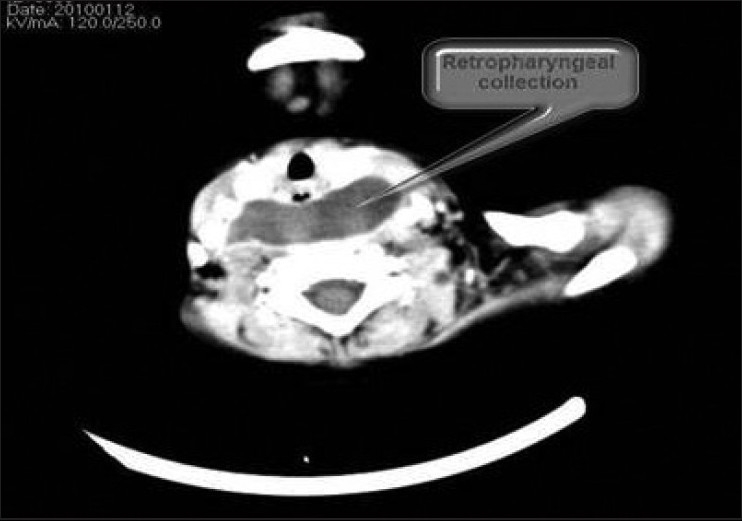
CT neck showing prevertebral collection
